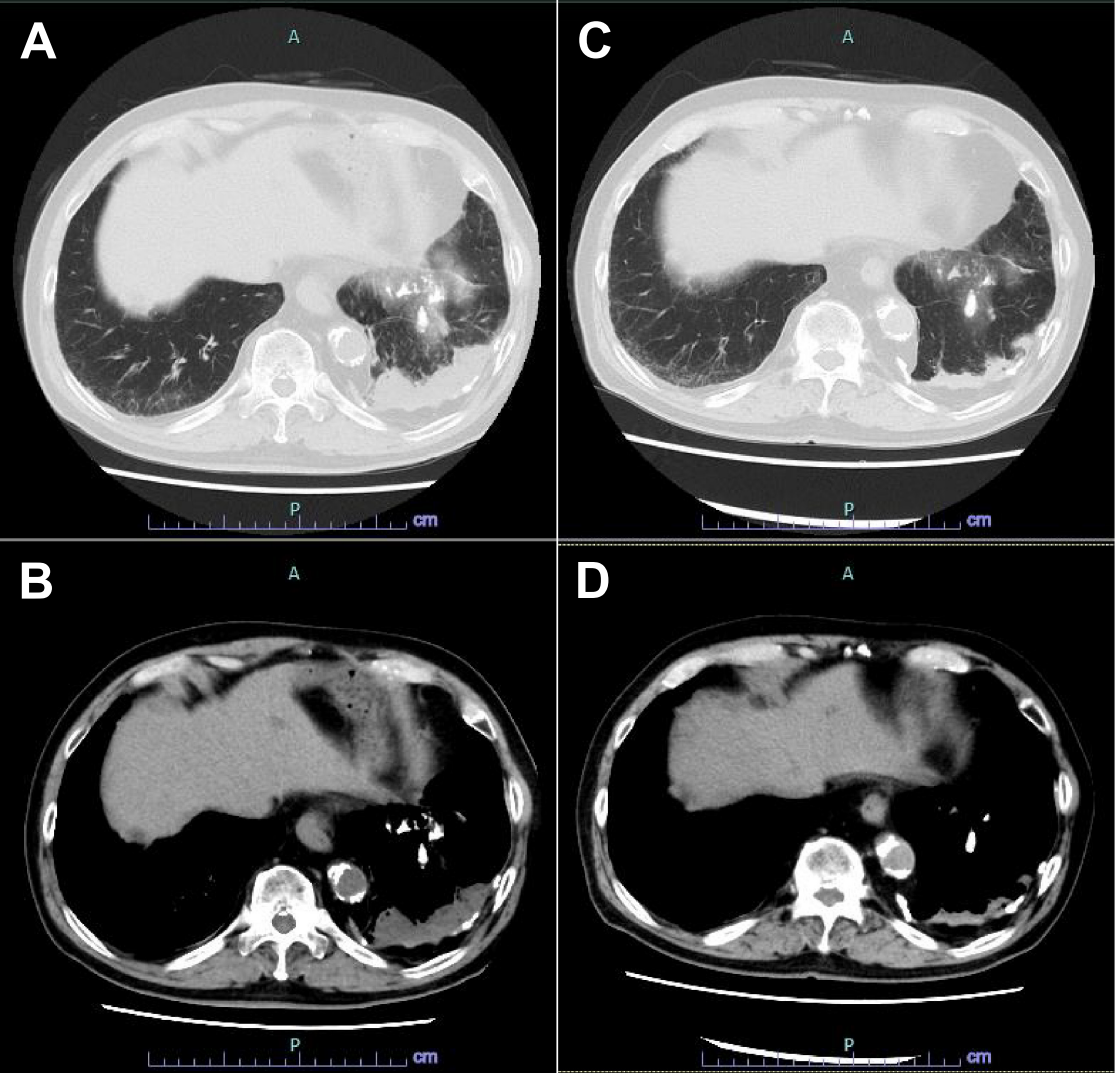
Clinical course of computed tomography findings. An exaggerated consolidative shadow around the rounded atelectasis appeared after atezolizumab treatment (A, B), which cleared after steroid treatment (C, D).
